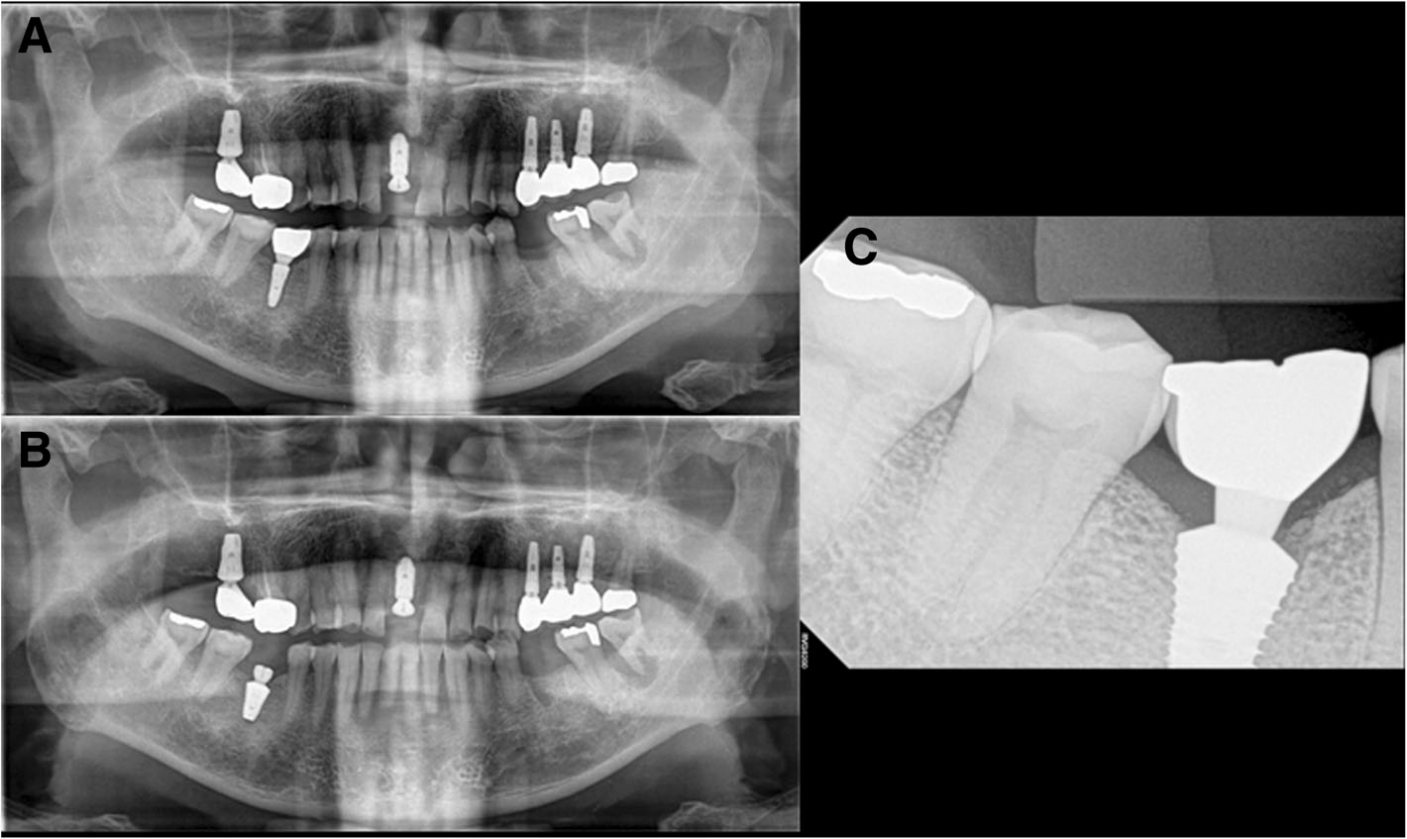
M3, radiographs a #46i second failure, preoperative panoramic radiograph, b #46i, third installation, c #46i, prosthesis delivery
